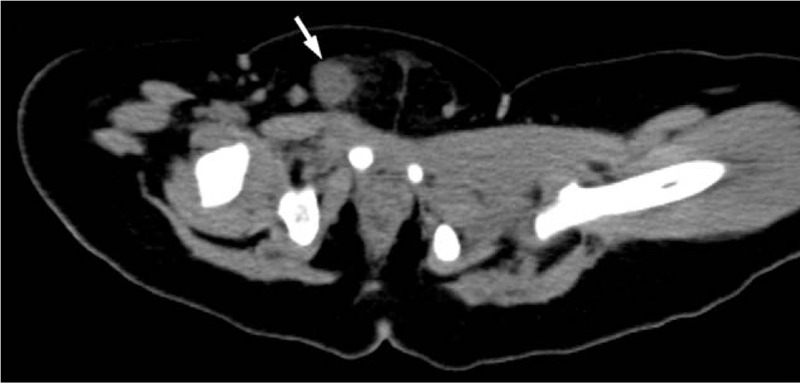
CT examination was performed. No radiopaque FB were found in CT views but the hypertrophic spermatic cord (arrow). CT = computer tomography, FB = foreign body.
